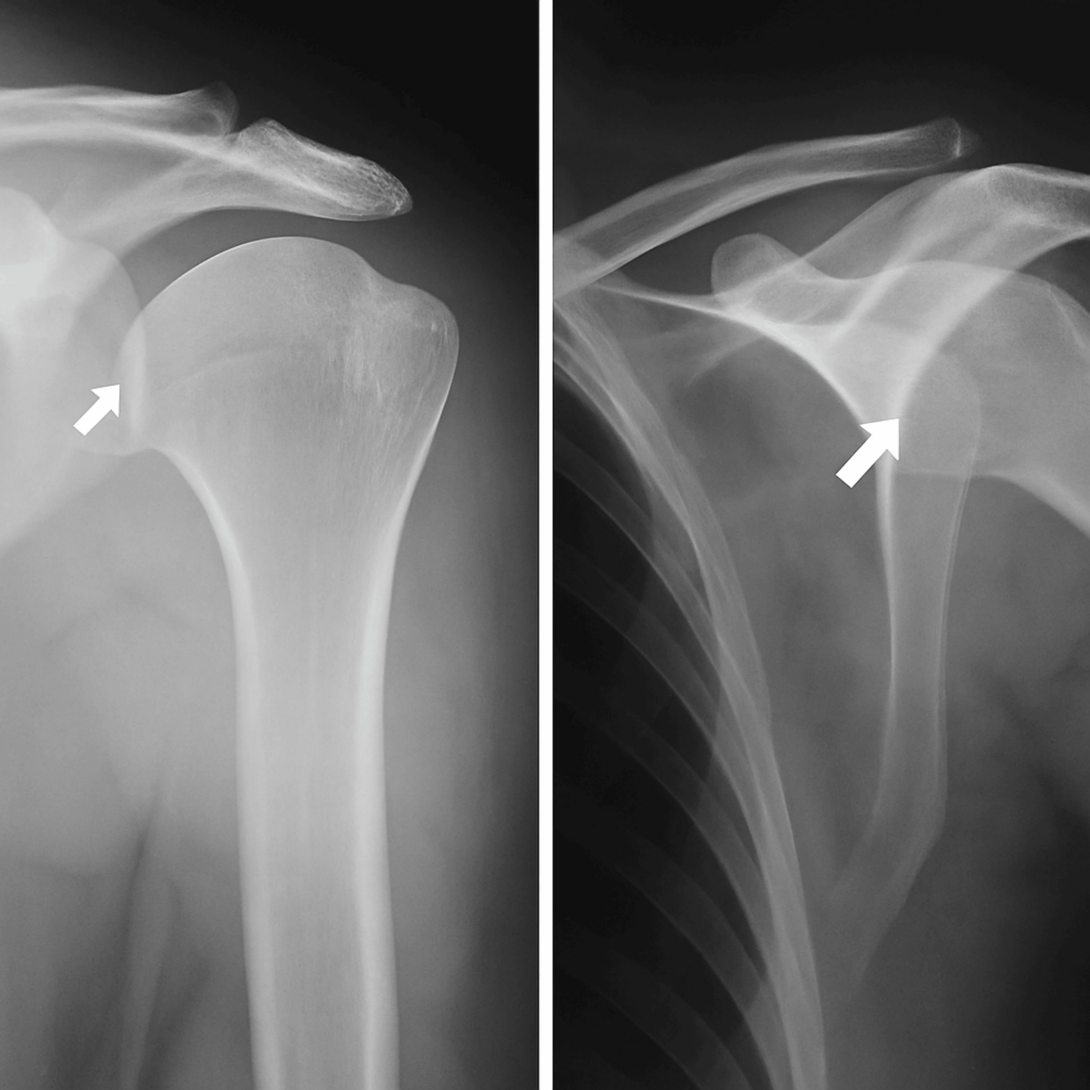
Large Hill-Sachs lesion on the posterosuperior aspect of the humerus X-ray of the left shoulder reveals a wedge-shaped, large Hill-Sachs lesion on the posterosuperior aspect of the humeral head (arrow).
